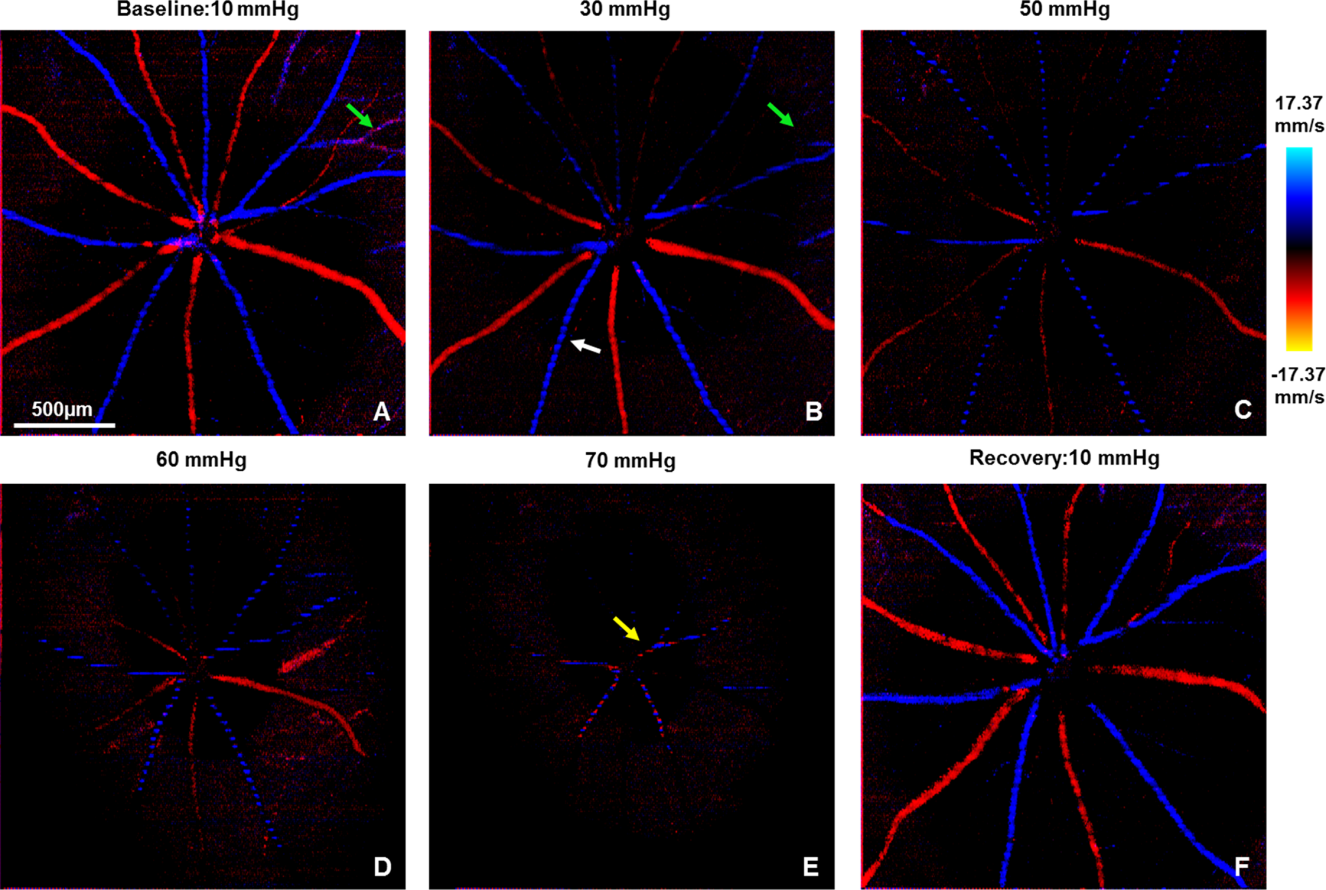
Representative *en-face* max projected Doppler OCT images in response to different IOP levels. Blue and red color represent arteries and veins, respectively. White arrow indicates the pronounced cardiac pulsation on the retinal arterial flow. Yellow arrow indicates that the cardiac pulsation caused retinal flow direction change at highly ischemic level IOP. Green arrows: vanished visualization of small vessels at 30 mmHg IOP. Scale bar = 100μm.
